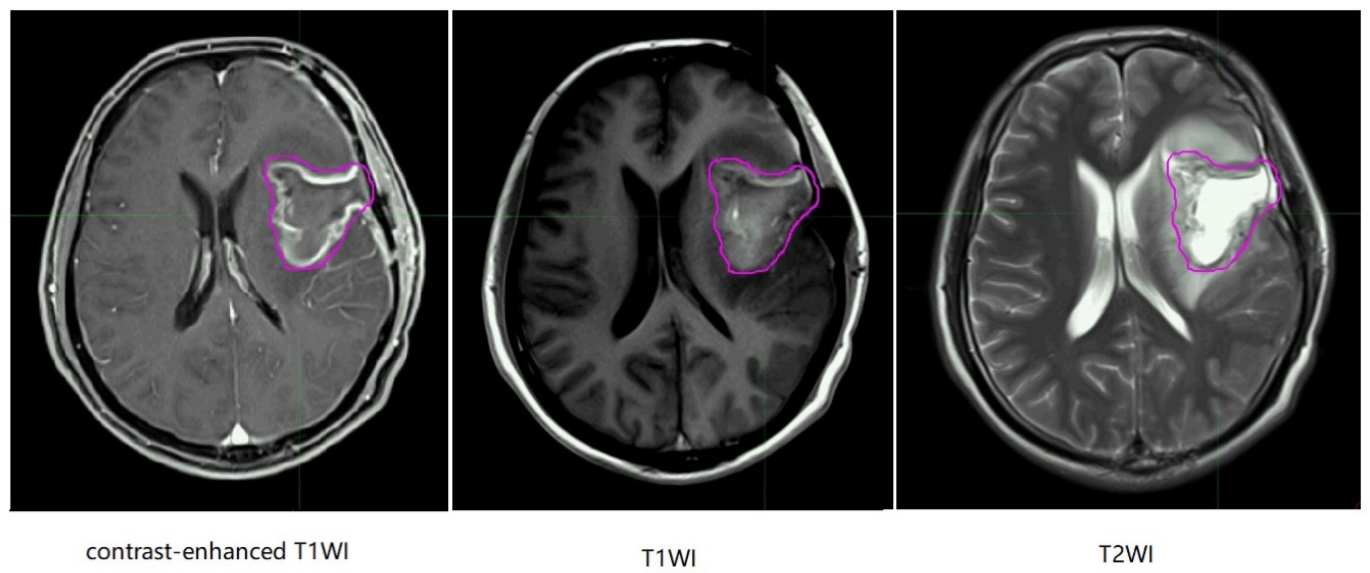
Representative non-recurrence patient's regular brain MRI images, the purple line represents gross tumor volume. T1WI, T1 weighted image; T2WI, T2 weighted image.
